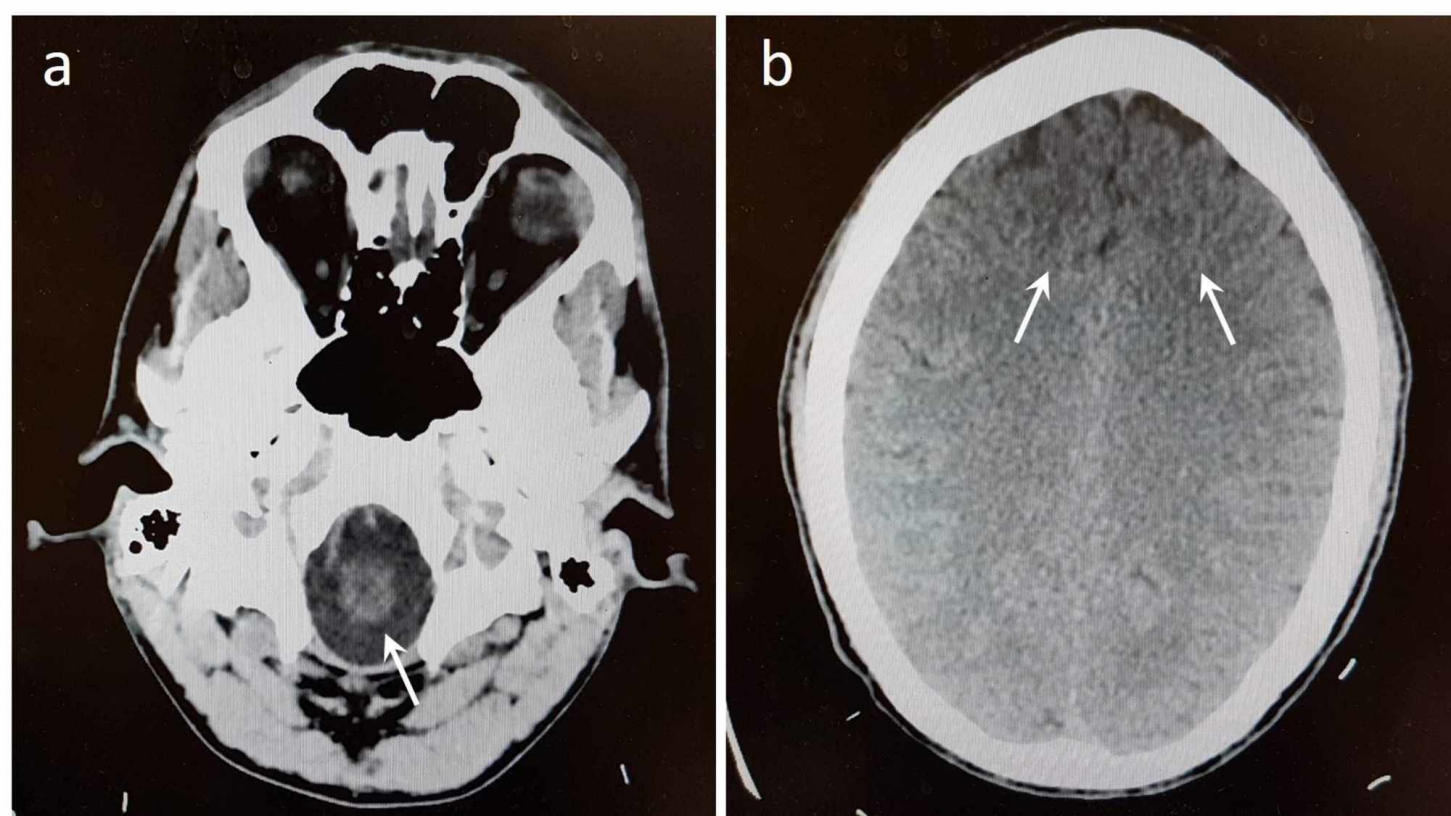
Magnetic resonance imaging (MRI) of the brain without contrast. MRI of the brainstem (a) and cortical regions (b) shows no lesions or structural abnormalities that would influence addictive behavior (arrows).
